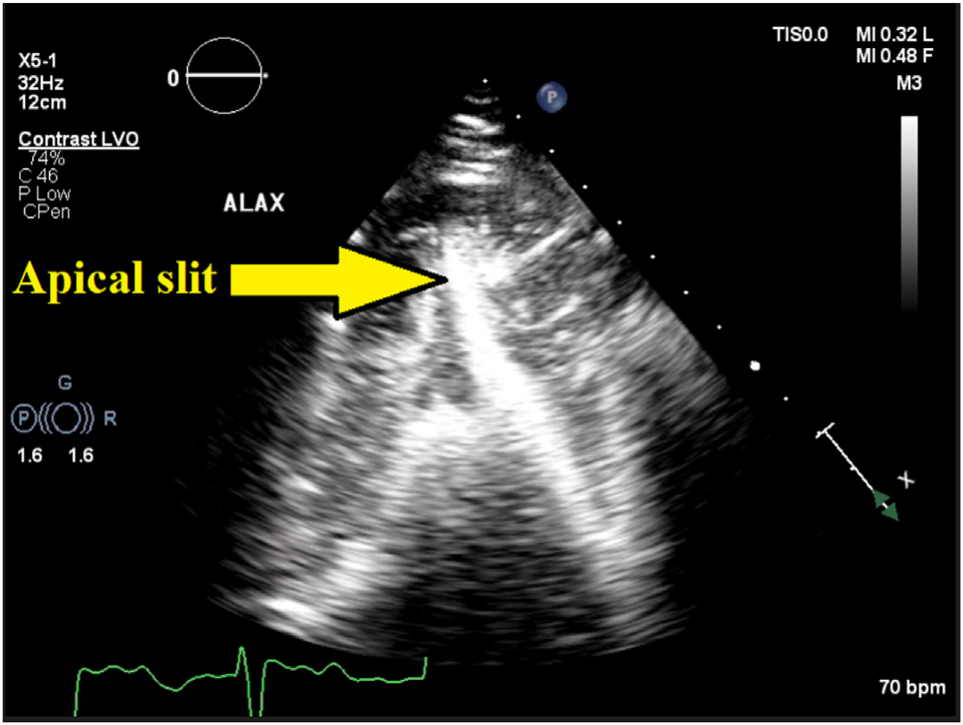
Two-dimensional TTE, apical long-axis view in diastole after administration of an ultrasound-enhancing agent, demonstrates the slit-like LV cavity extending into the apical tip.
